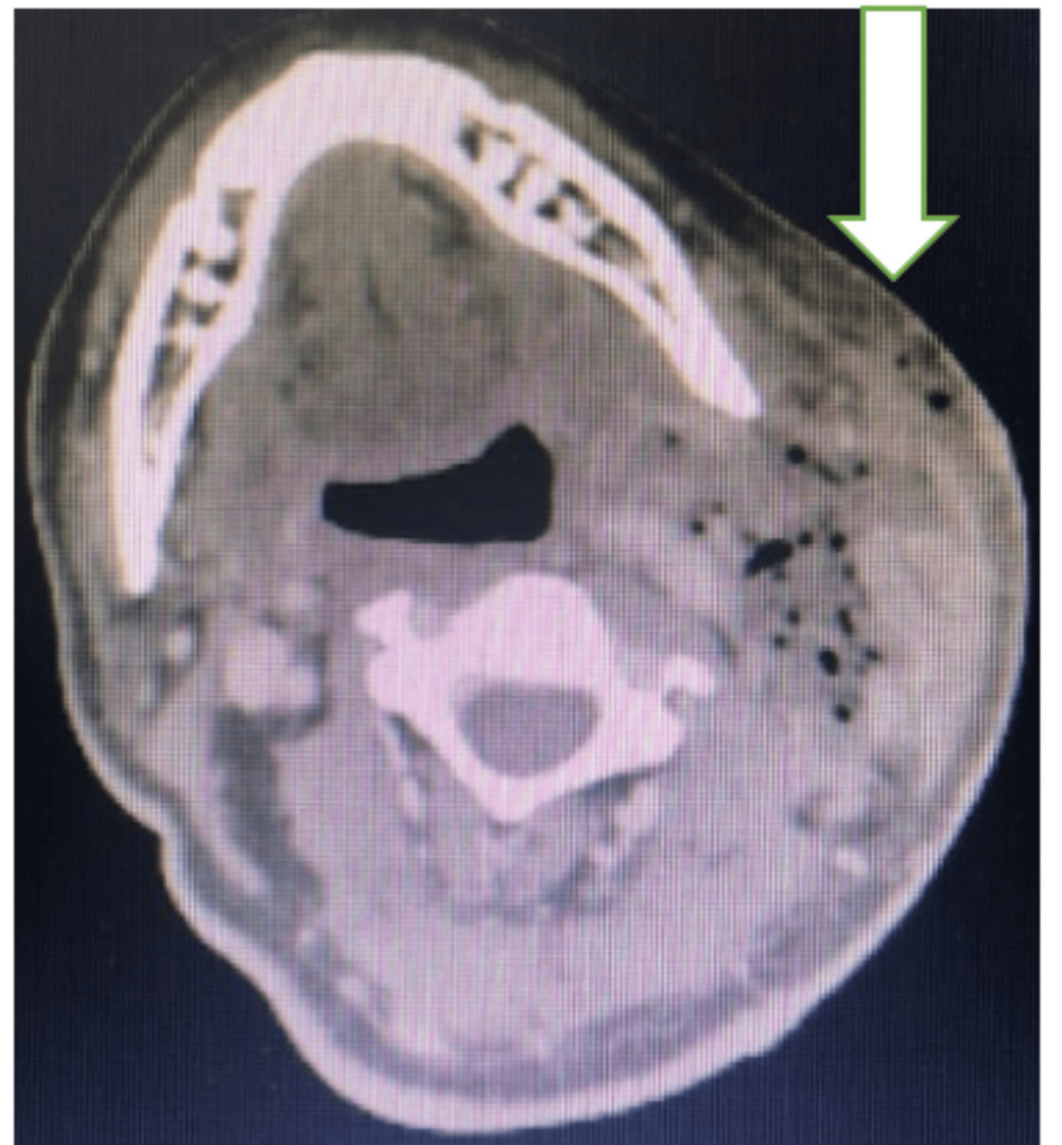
Contrast-enhanced CT showing extensive necrotizing inflammatory changes involving the left parotid, parapharyngeal, submandibular, and carotid spaces. The white arrow indicates the necrotic collection lateral to the sternocleidomastoid muscle.
